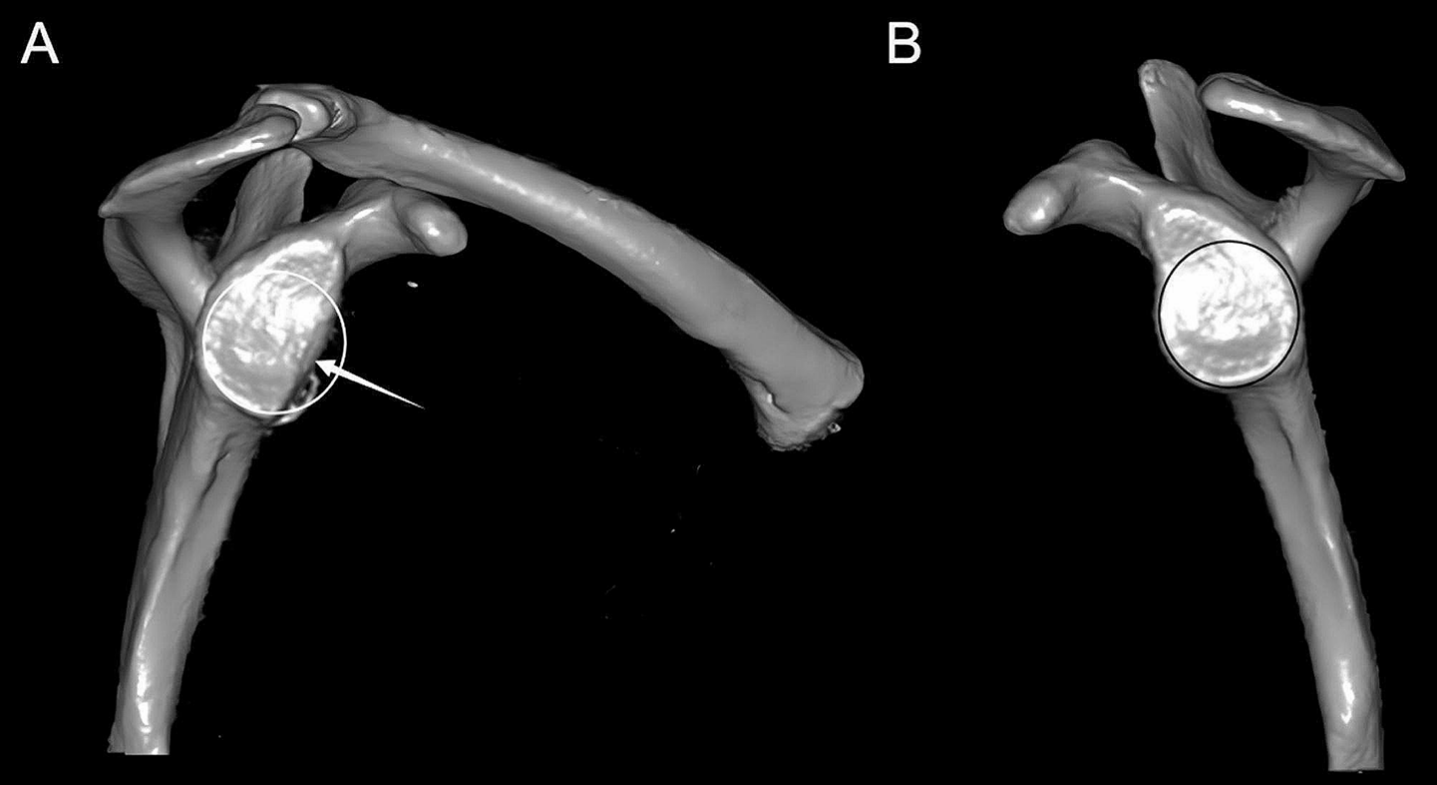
Preoperative 3D CT images of the affected side (A) and the healthy side (B) in the same case. The arrow indicates 25% anterior glenoid defect
